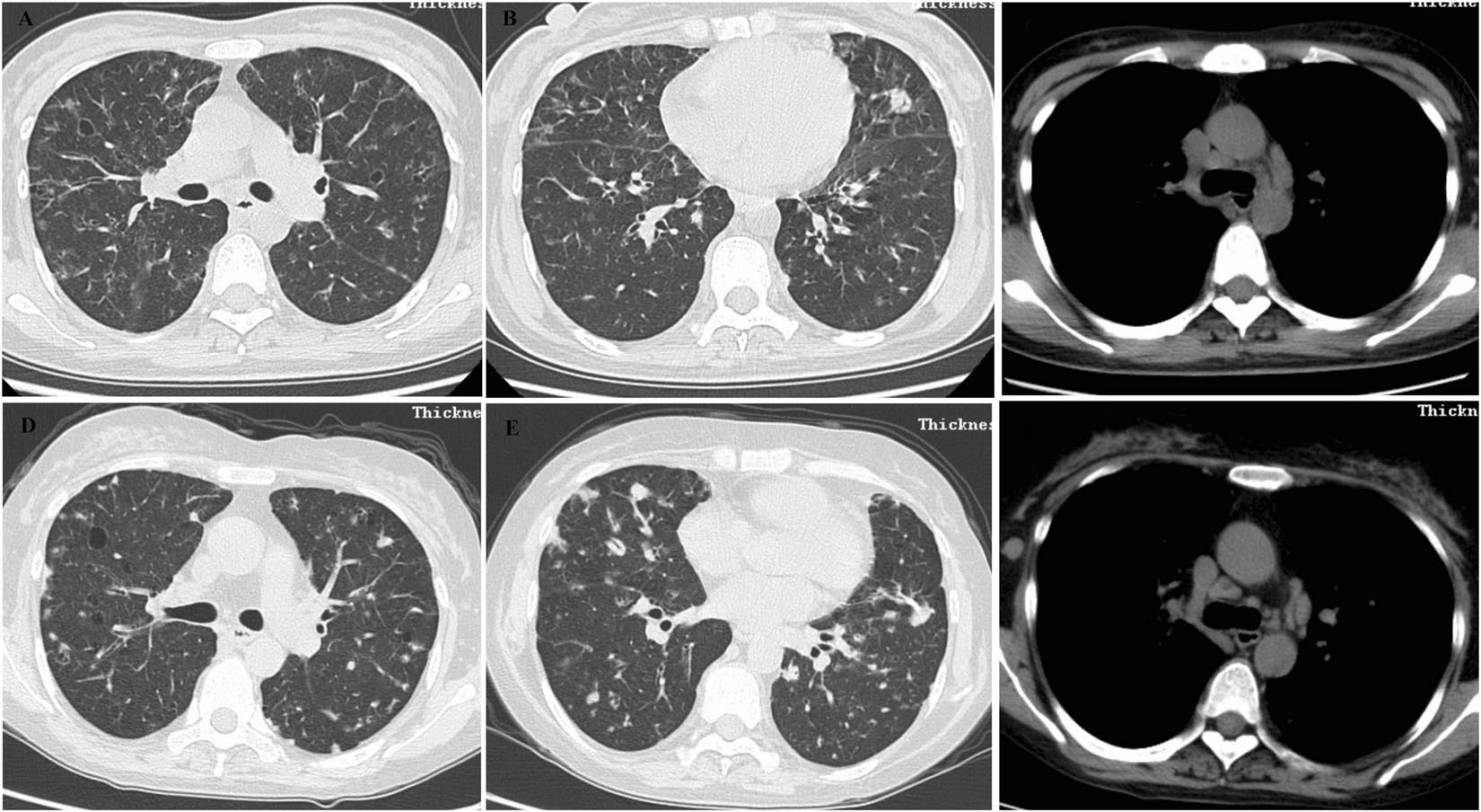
Case 3. (A–C) Chest CT (Mar 16, 2012) showed nonspecific nodules, interlobular septum thickening, diffuse patchy GGOs on lung window and mediastinal lymphadenopath on mediastinal window. (D–F) Chest HRCT (May 5, 2016) revealed increased bilateral multiple nodules and cysts, while decreased patchy GGOs compared with before on lung windows. The mediastinal lymphadenopaths were more and larger than before on mediastinal windows.
